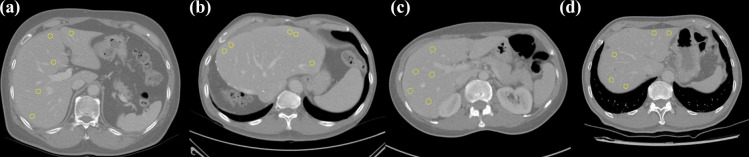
Sample homogeneous ROI extraction results on contrast-enhanced liver CT exams. The results were displayed for multi-vendor CT images acquired through (a) Scanner 1, (b) Scanner 2, (c) Scanner 3, and (d) Scanner 4, respectively.
